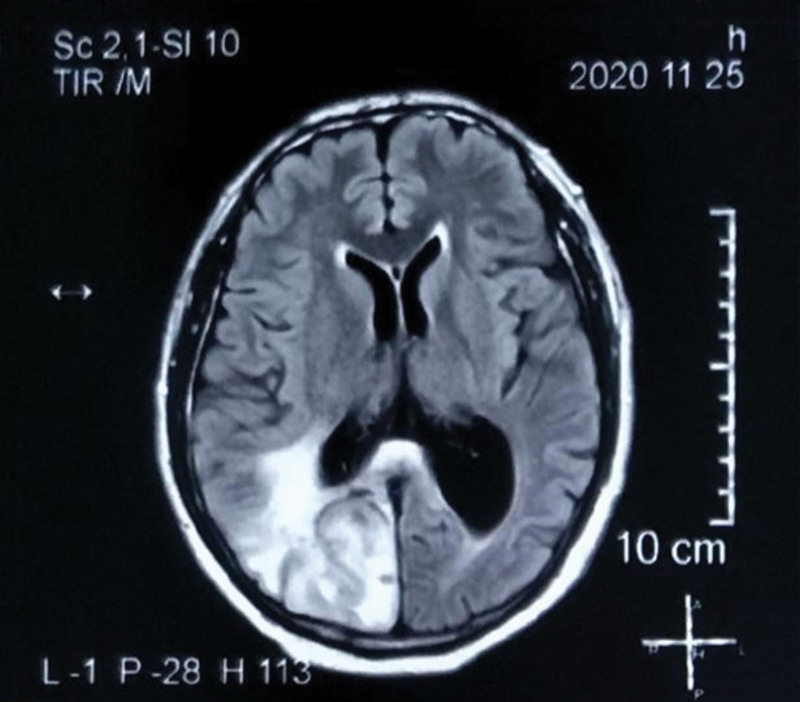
In November 2020, MRI indicated a smaller size of 2.7 × 1.2 cm of the lesion in the right occipital lobe. MRI = magnetic resonance imaging.
